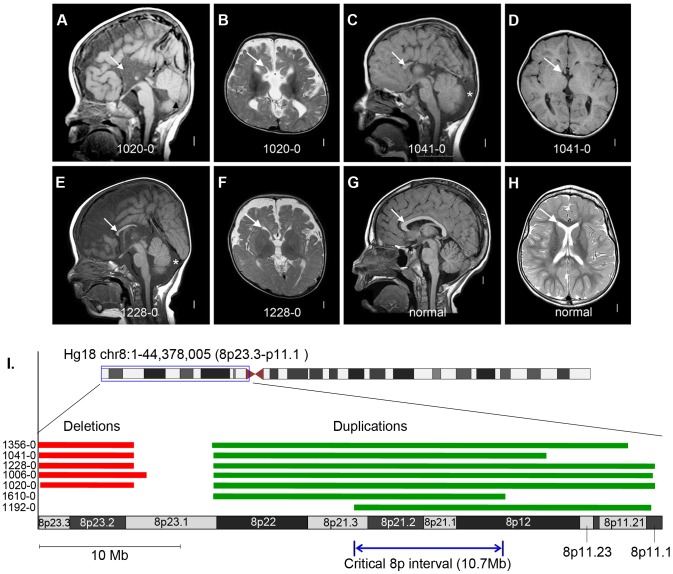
Brain magnetic resonance imaging and critical region analysis of the duplication-deletion 8p syndrome. Panels A–F show brain images of three select patients with ACC who had large *de novo* CNVs on chromosome 8p, and panels G–H show brain images of a normal individual. Midline sagittal images (panels A, C, E) demonstrate complete (panel A) or partial (panels C and E) agenesis of the corpus callosum indicated by white arrows. In panel A the black arrowhead points to mild hypoplasia and upward rotation of the cerebellar vermis, and in panels C and E the asterisks show enlarged cisterna magna below and behind the cerebellum. Axial images of patients at the level of the thalamus demonstrate enlarged extra-axial fluid over the frontal lobes and mildly enlarged 3rd ventricles (panels B and F). In the patient with complete agenesis, the lateral ventricles are also mildly enlarged and widely separated (panel B). Panel I shows the genomic locations of the chromosome 8p CNVs in seven patients with ACC, with patients 1610-0 and 1192-0 being instrumental in defining the 10.7 Mb critical region (Hg18 chr8:22609566-33311183). Table S10 lists the clinical features of all seven patients.
